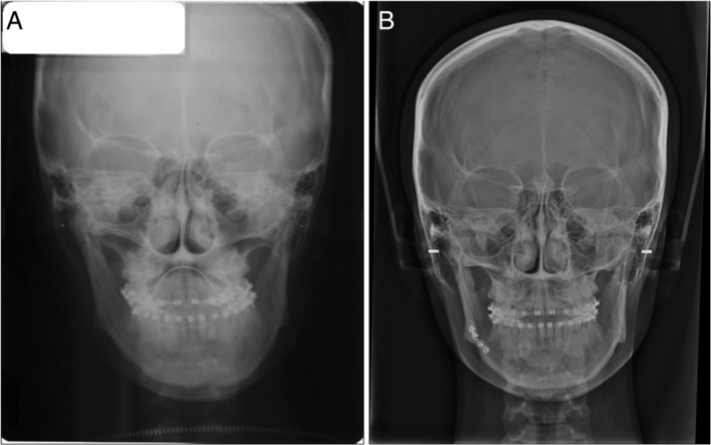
Radiographic findings of case 1: a pre-operation and b post-operation
